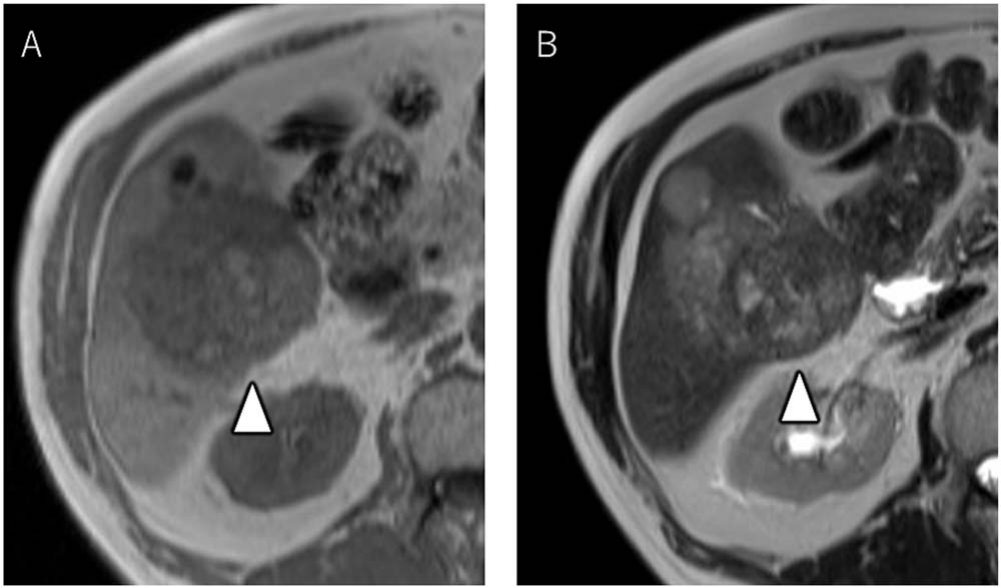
Magnetic resonance imaging showing multiple nodular lesions in liver S6/5 with low signal intensity on T1-weighted images (A), and mosaic-like high signal intensity on T2-weighted images (B).
